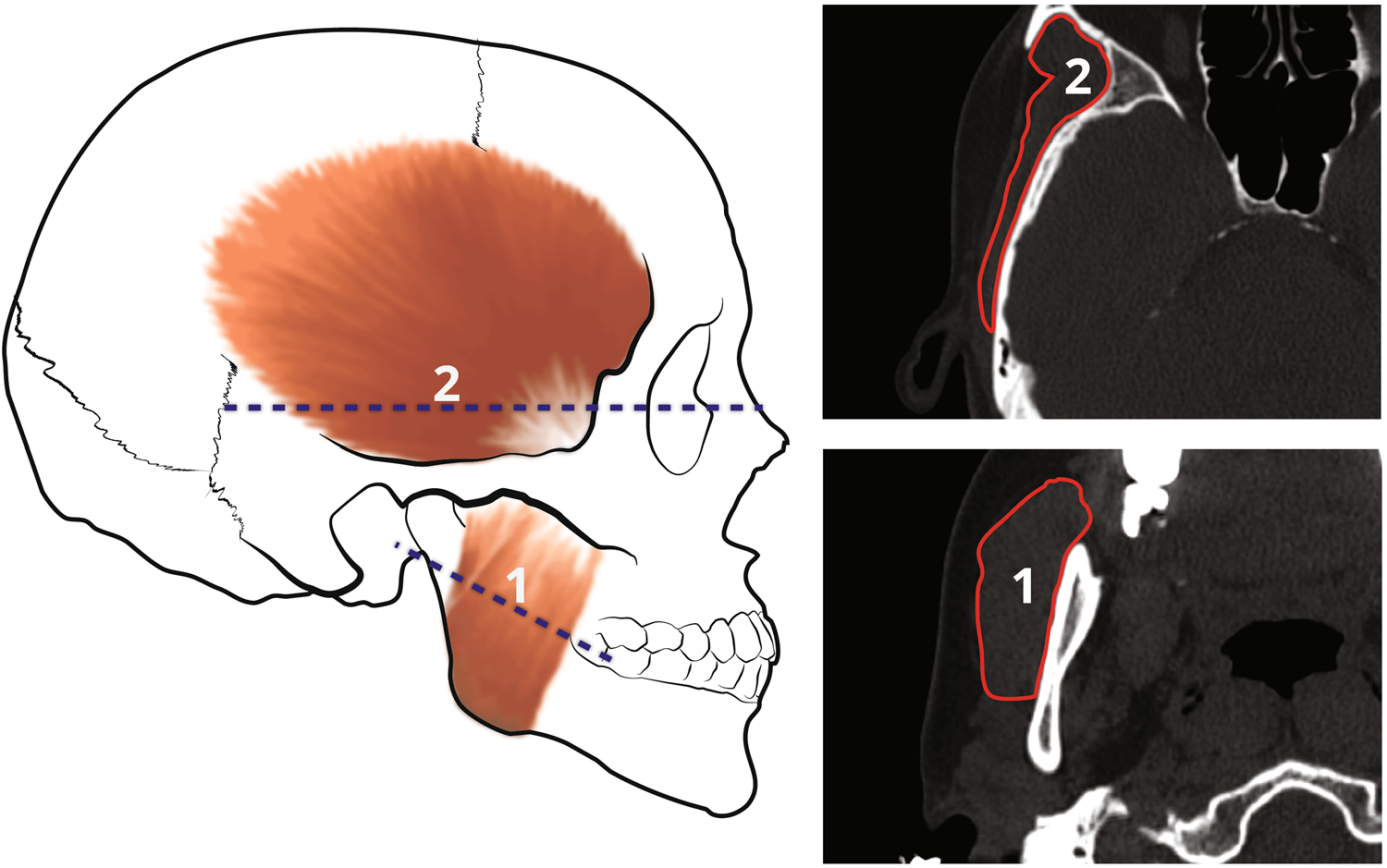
Muscle cross-sectional area measurement following Weijs and Hillen^28^. Masseter (1) area was estimated by tracing it on the CT scan sectioned 3 cm ventro-cranially to the jaw angle, 30° relative to the Frankfurt horizontal plane. Temporalis (2) area was measured one cm cranially to the zygomatic arch, parallel to the Frankfurt horizontal plane.
